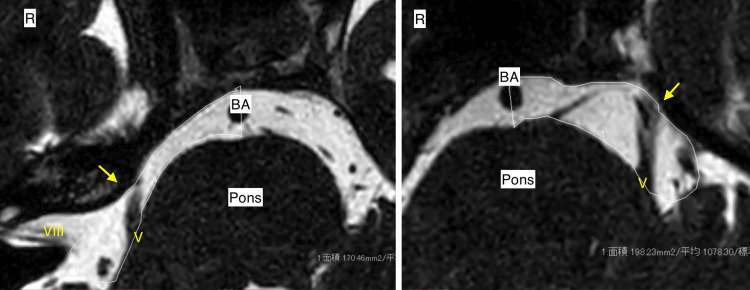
MRI T2-weighted images. MRI scan demonstrates a very narrow space around the right cerebellopontine cistern with a bulky shape of the petrous bone. MRI: magnetic resonance imaging; BA: basilar artery; V: cranial nerve V; VIII: cranial nerve VIII
